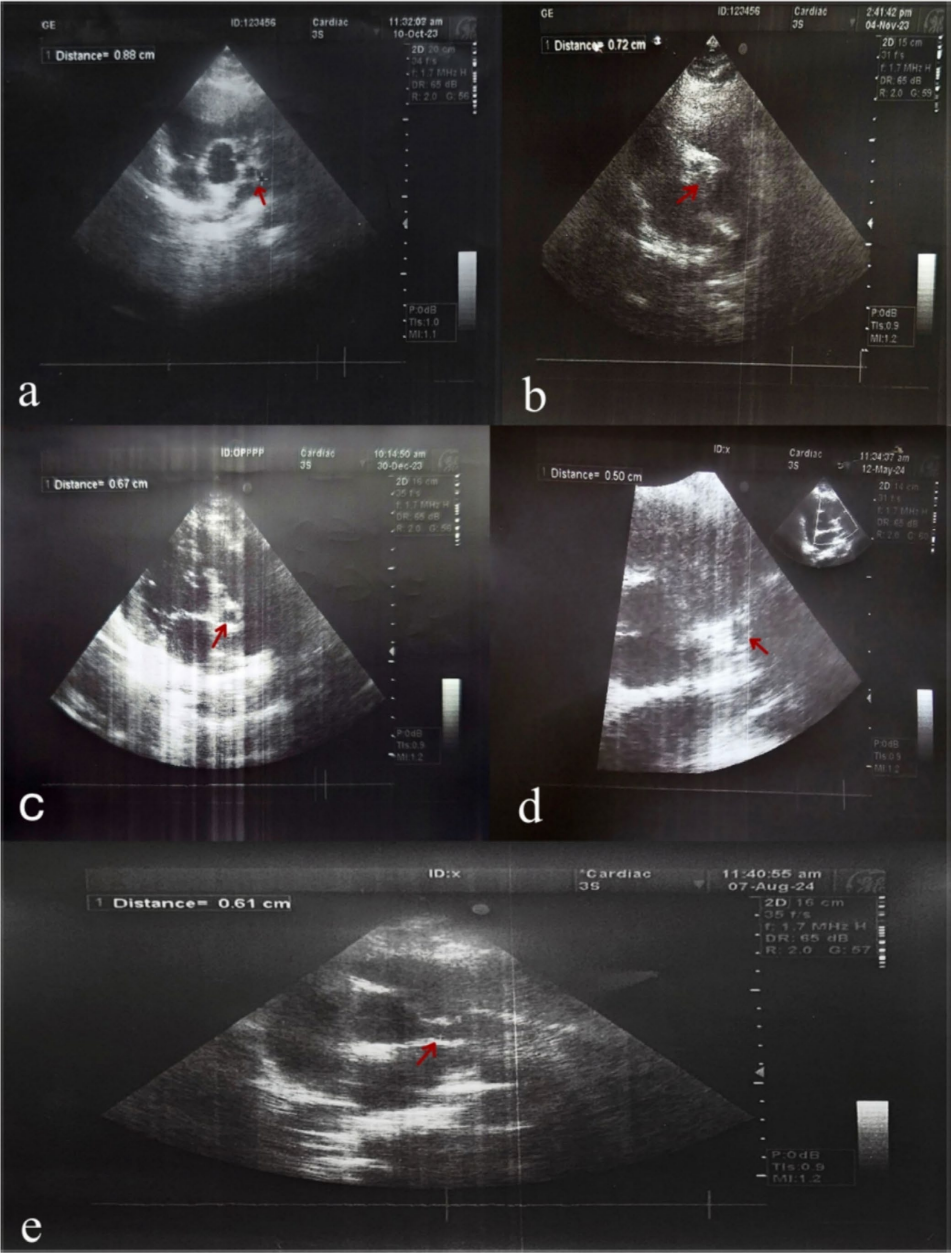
Serial echocardiography follow‐up. Echocardiographic measurements showing changes in the left coronary artery aneurysm over time, with regression from 8.8 mm to 5.0 mm at 7 months, followed by an increase to 6.1 mm at 10 months.
